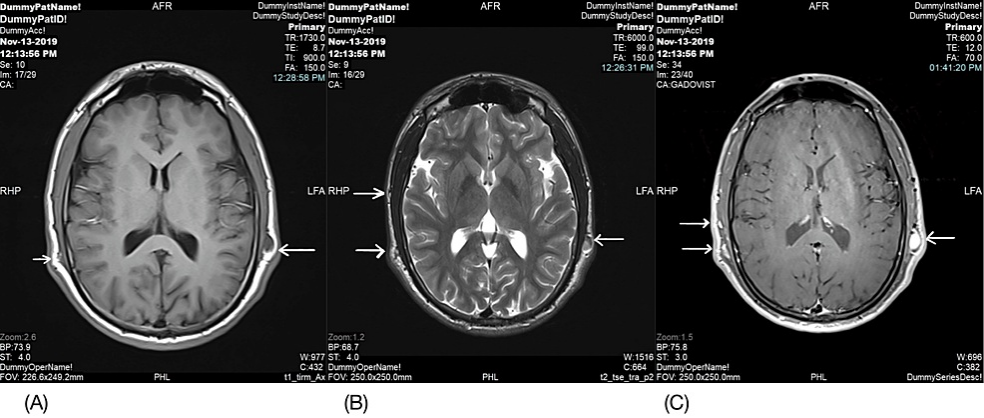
Magnetic resonance imaging of the brain unveiled multiple subcutaneous neurofibromas (arrows)
